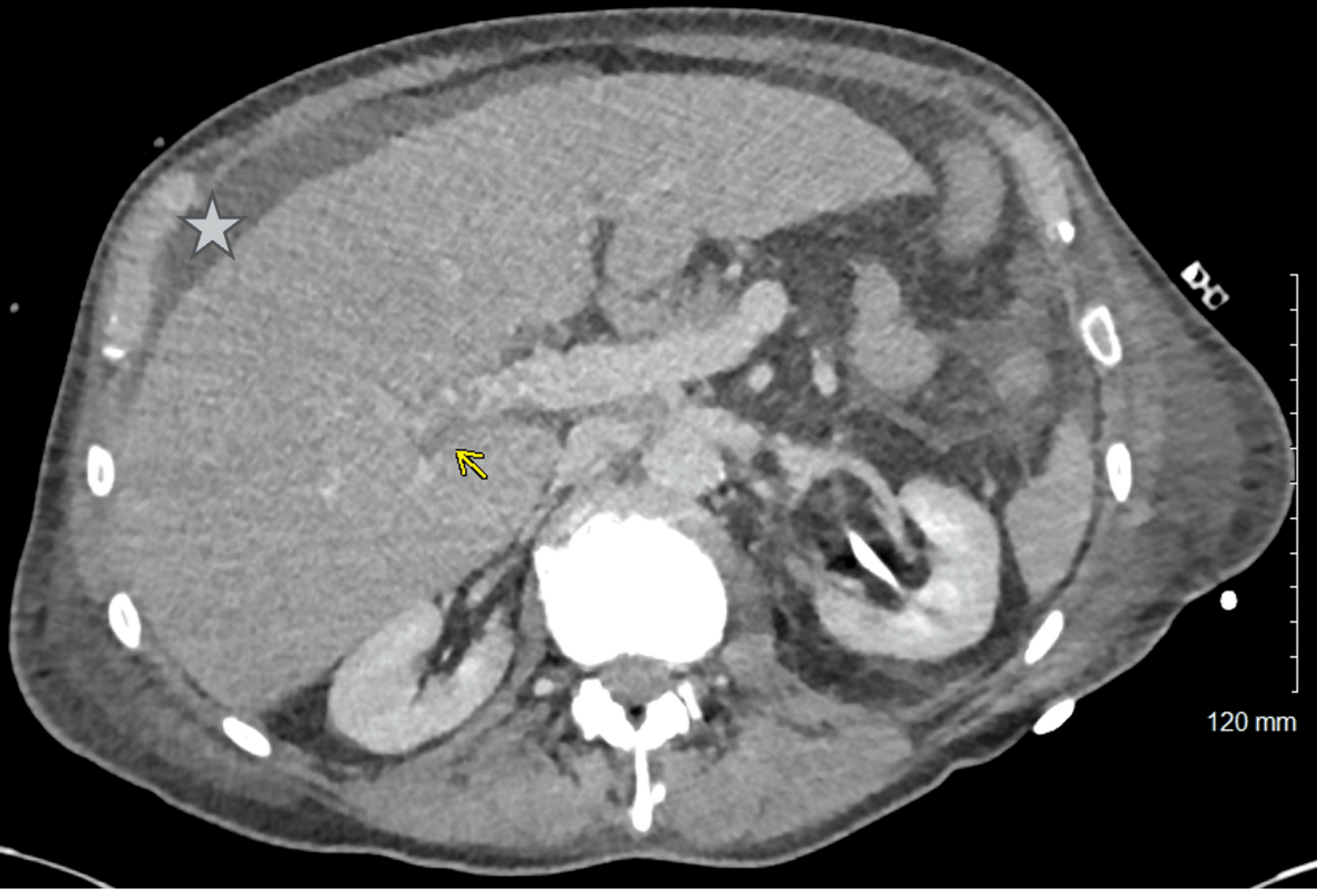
CT abdomen pelvis showing portal vein thrombosis The arrow points to portal vein thrombosis. The star indicates ascites.
